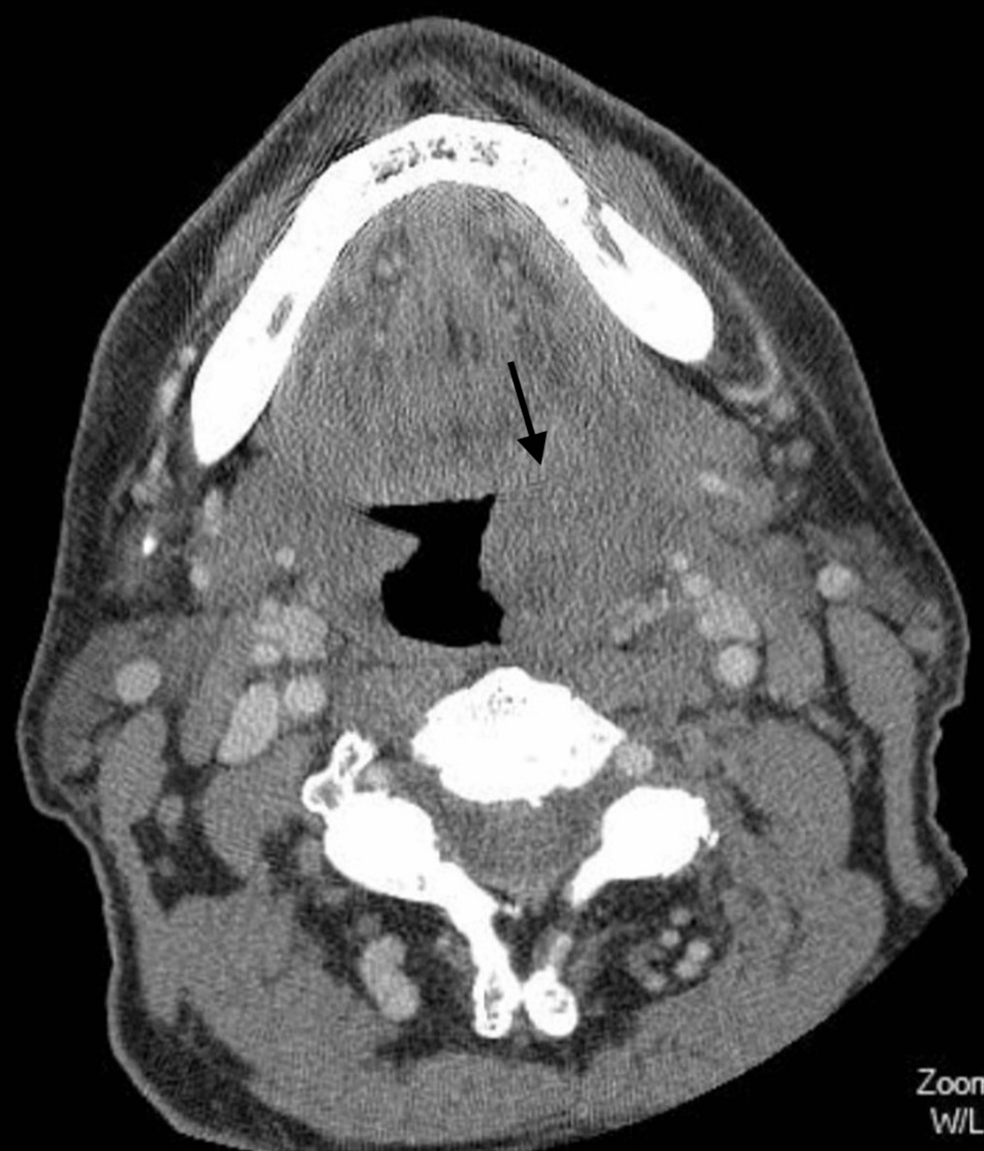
Initial CT scan with IV contrast of left palatine tonsillar mass before undergoing outpatient antibiotic treatment.
